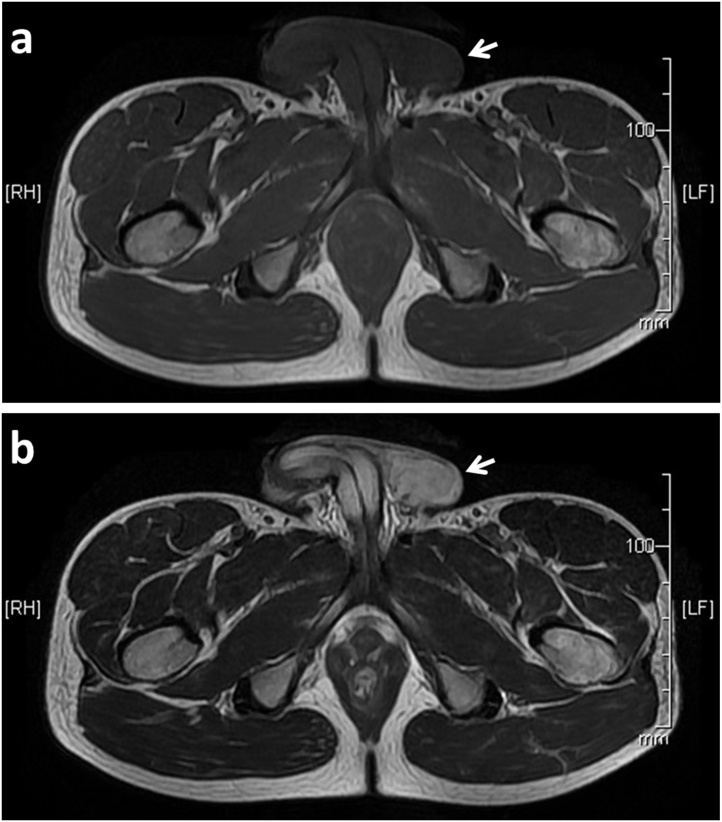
MRI showed a 50 mm low intensity well-demarcated lesion on T1-weighted image(a) and a high intensity lesion on T2-weighted image (b).
